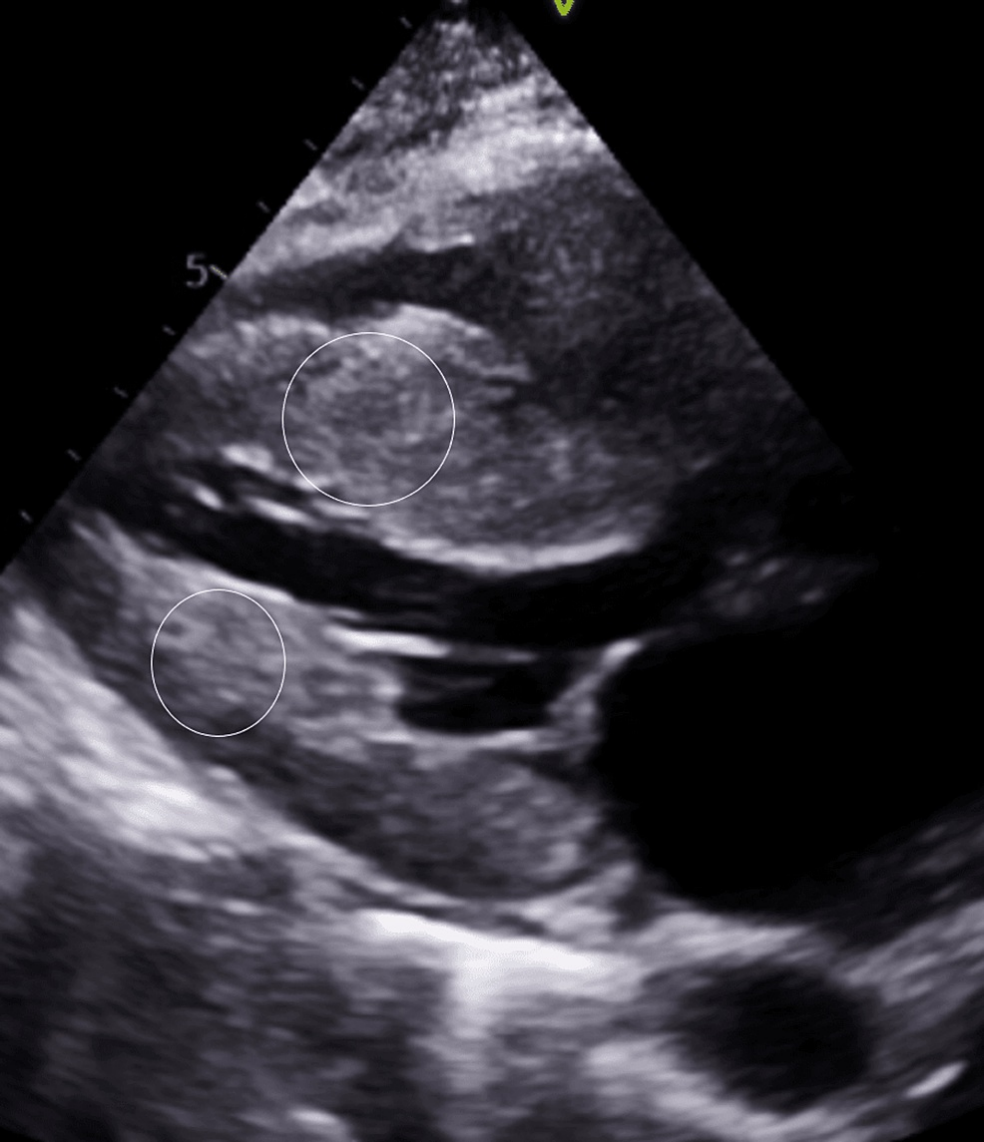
Parasternal long-axis echocardiogram view. Parasternal long-axis echocardiogram view showing increased left ventricular wall thickness (circles).
